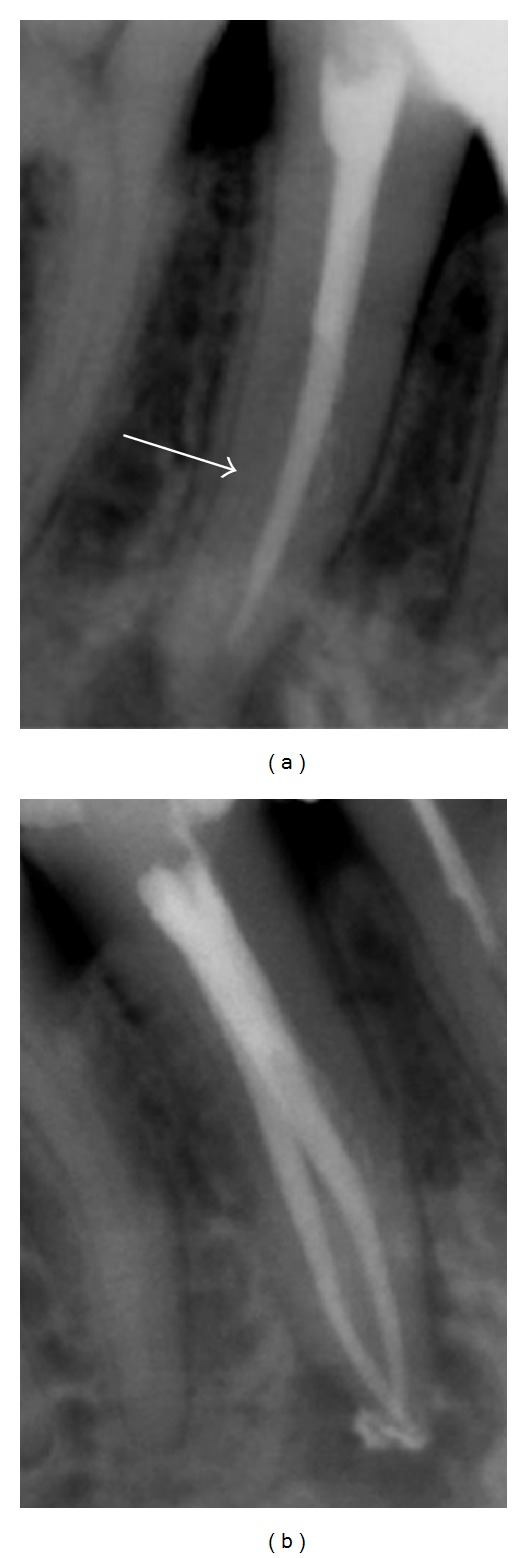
(a) A mandibular second premolar with a missed lingual canal seen distal to the obturated straight buccal canal (white arrow), seen as a mid-root off-shoot at a sharp angle, visualized as a lower letter case “h.” (b) Postretreatment radiograph with obturation of both buccal and lingual canals.
